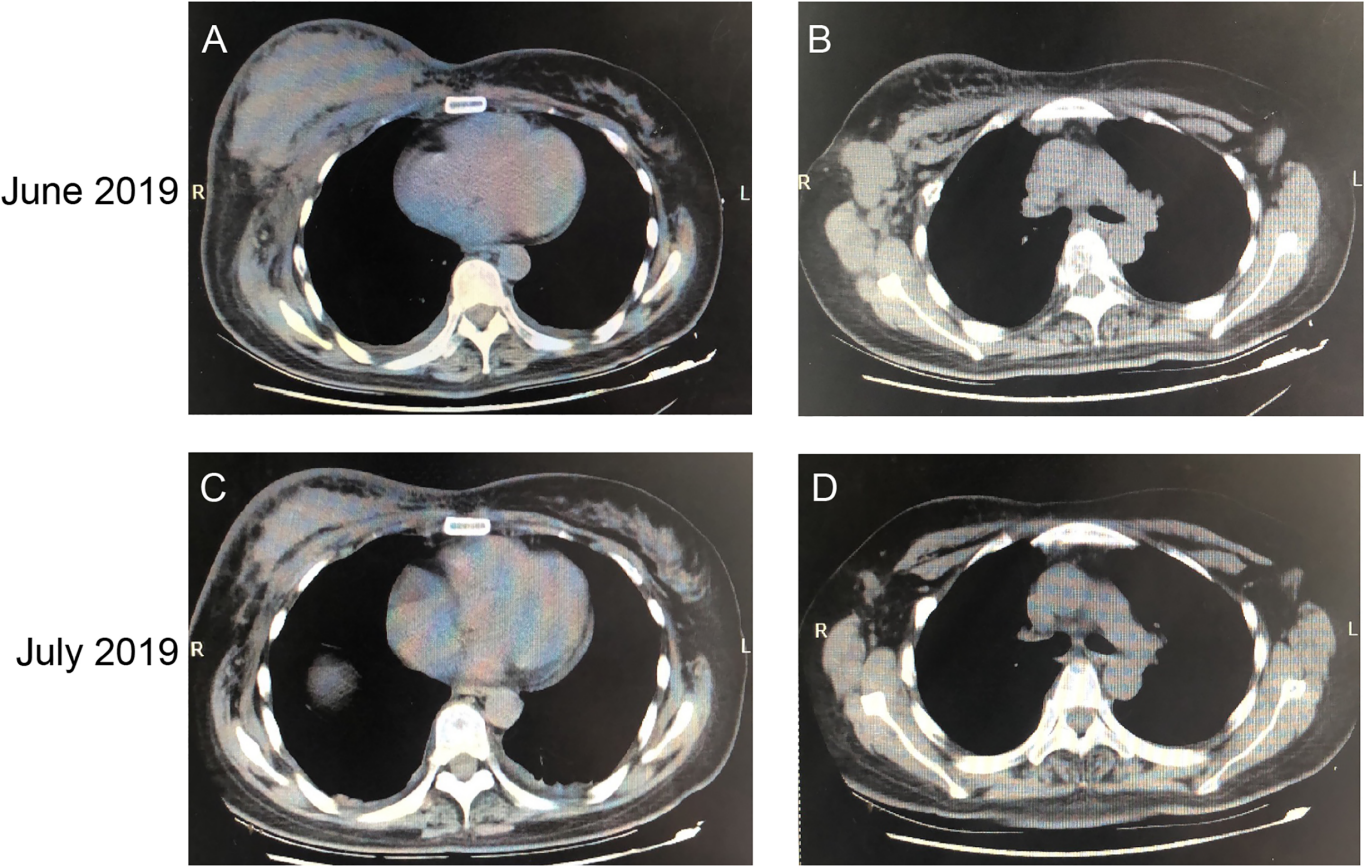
CT Imaging of the breast and lymph node before and after pyrotinib treatment. CT scans before the administration of pyrotinib (images A, B) and after one month of treatment (images C, D), showing the tumor mass and lymph node.
